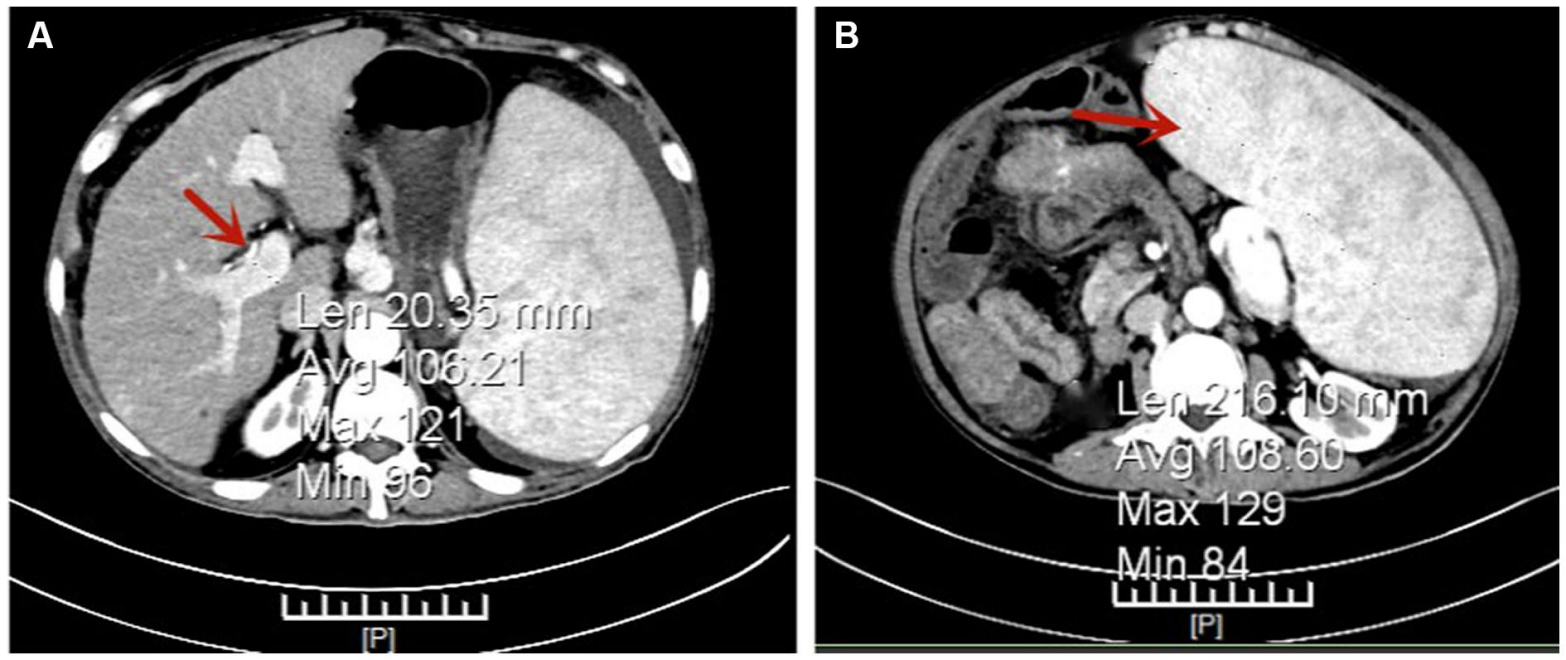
Portal Vein Computed Tomography Venography (CTV) Indicating Pulmonary Hypertension (PH). Panel (A) shows the main portal vein with a width of approximately 20 mm, as highlighted by the arrow. Panel (B) demonstrates a significantly enlarged spleen, as indicated by the arrow.
